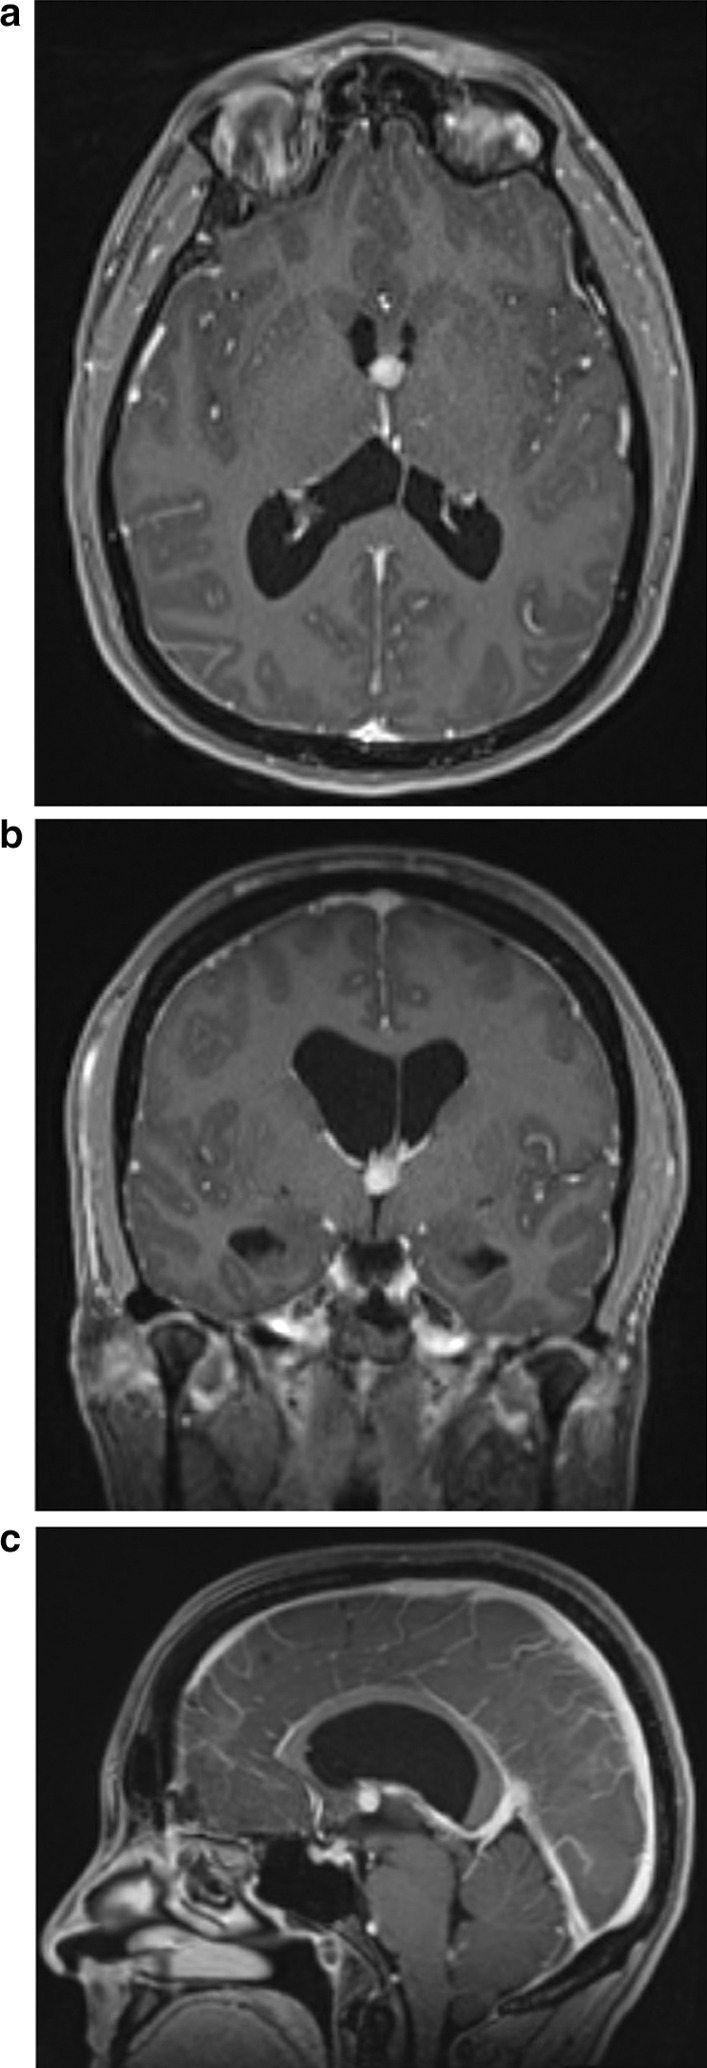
Preoperative axial (a), coronal (b), and sagittal (c) T1 postcontrast magnetic resonance imaging demonstrating a colloid cyst at the foramen on Monroe. Note the dilated lateral ventricles and bowing of the corpus callosum suggestive of chronic hydrocephalus
